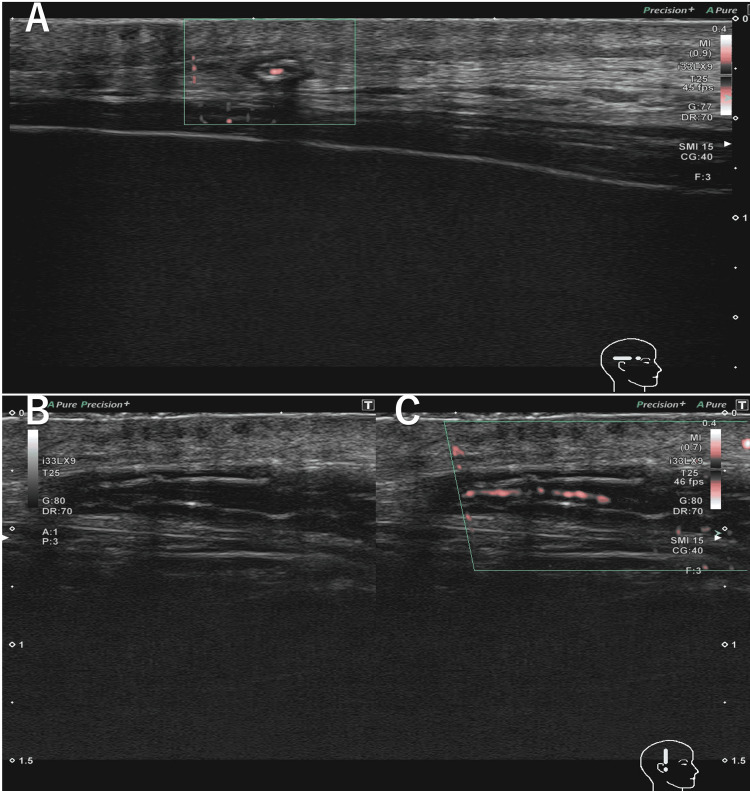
Temporal artery (TA) ultrasonography A. Color Doppler in the short-axis view (GG: 77 dB, CG: 40 dB, Frequencies: 15 MHz, PRF: 156 Hz) B. B-mode in the long-axis view (GG: 80 dB) C. Color Doppler in the long-axis view (GG: 80 dB, CG: 40 dB, Frequencies: 15 MHz, PRF: 156 Hz) TA ultrasonography shows right common temporal artery hyperechoic wall thickening circumferentially. CG: color gain, GG: grey gain, PRF: pulse repetition frequency
